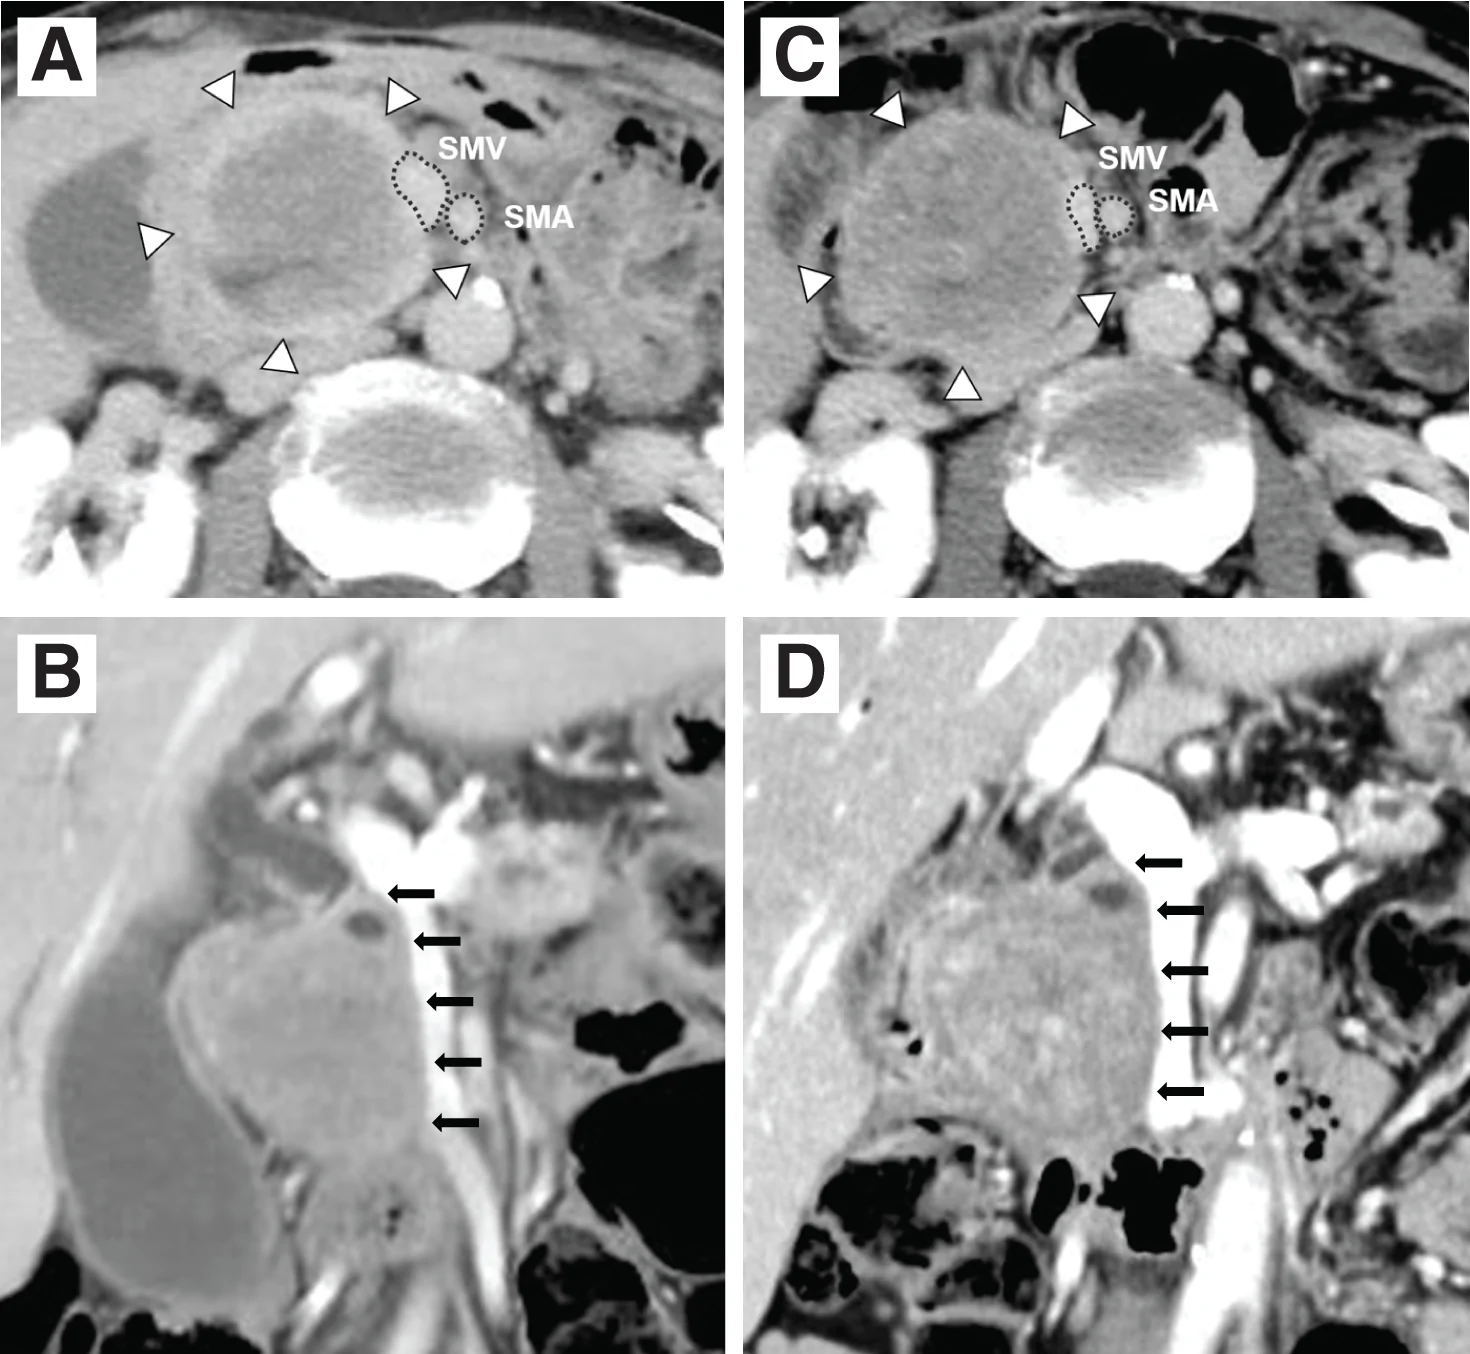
Contrast-enhanced CT before and after systemic chemotherapy. (A) Axial contrast-enhanced CT before systemic chemotherapy shows a 60-mm mass in the pancreatic head abutting the SMV–PV axis. (B) Coronal contrast-enhanced CT before systemic chemotherapy shows long-segment contact between the tumor and the SMV–PV axis, measuring approximately 50 mm in the craniocaudal direction. (C) Axial contrast-enhanced CT after systemic chemotherapy shows a modest decrease in tumor size to 50 mm, with persistent long-segment contact with the SMV–PV axis. (D) Coronal contrast-enhanced CT after systemic chemotherapy shows residual long-segment contact with the SMV–PV axis. The contact length on coronal images remained approximately 50 mm, showing no substantial interval change. The imaging response to systemic chemotherapy was classified as stable disease according to RECIST version 1.1. SMA, superior mesenteric artery; SMV, superior mesenteric vein; PV, portal vein; RECIST, Response Evaluation Criteria in Solid Tumors
